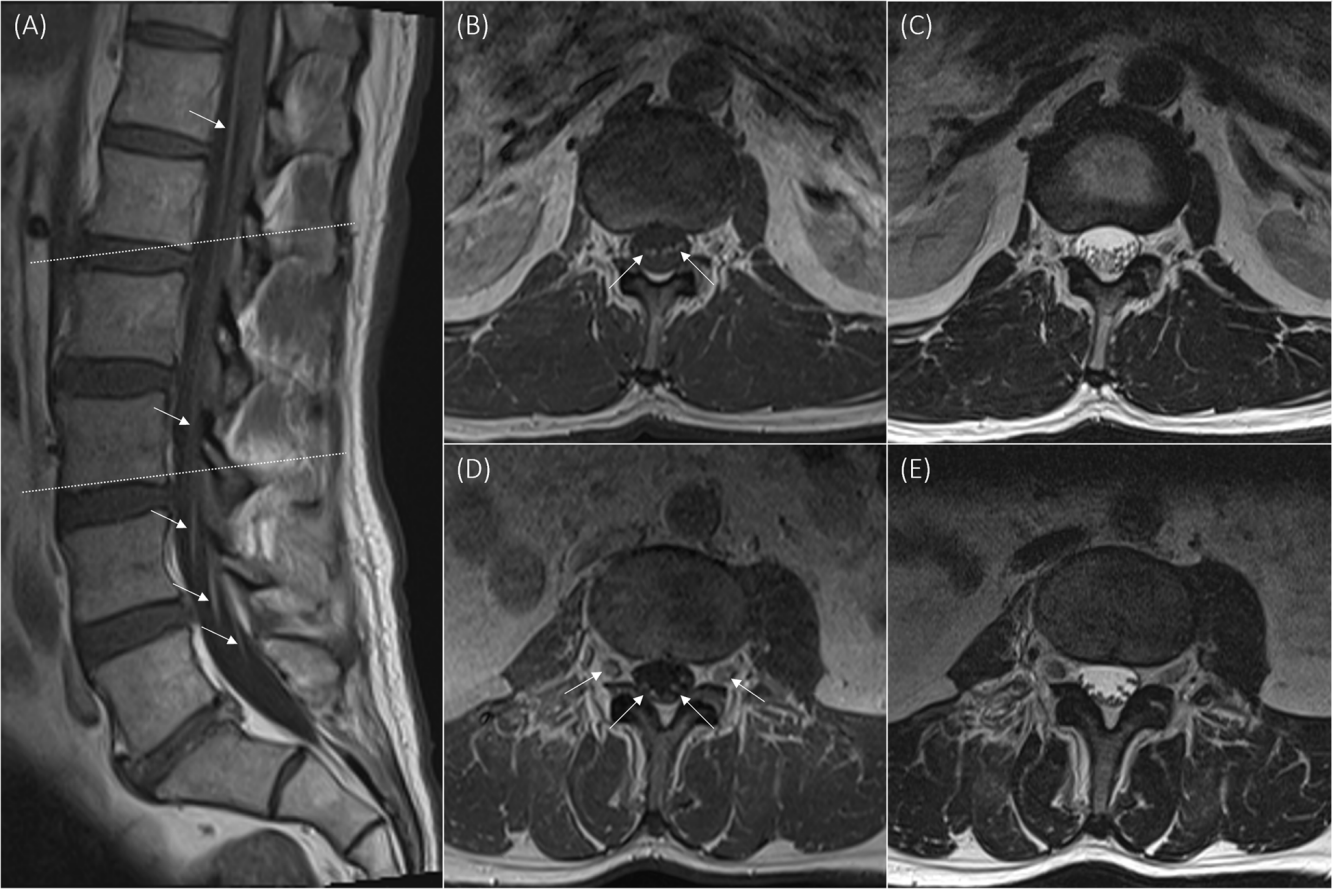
MRI lumbar spine: T1 post-gadolinium sagittal (A) and axial (B, D) views showing smooth enhancement of ventral nerve roots (arrows), with corresponding T2 axial (C, E) views. Panel A, upper dashed line corresponds to axial slices B and C, lower dashed line corresponds to axial slices D and E. MRI = magnetic resonance imaging
